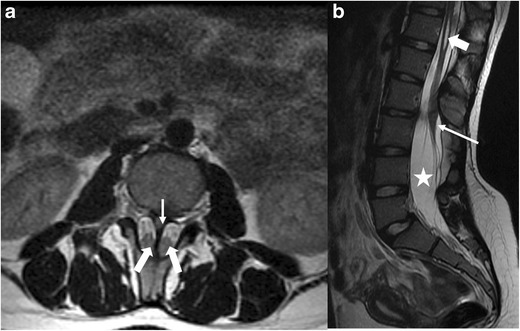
a-b: Split cord/diastematomyelia in 15 year old: Axial (*a*) and sagittal T2-weighted (*b*) MR images of the lumbar spine reveals an osseous septum (*thin arrow* on *a*) dividing the spinal cord into symmetric halves (*thick, block arrows* on *a* ). Notice that the cord is low-lying and posteriorly positioned within the spinal canal (*thin arrow* on *b*) and contains a syrinx (*block arrow* on *b* ). Associated dural ectasia (*star* on *b*) is also seen
